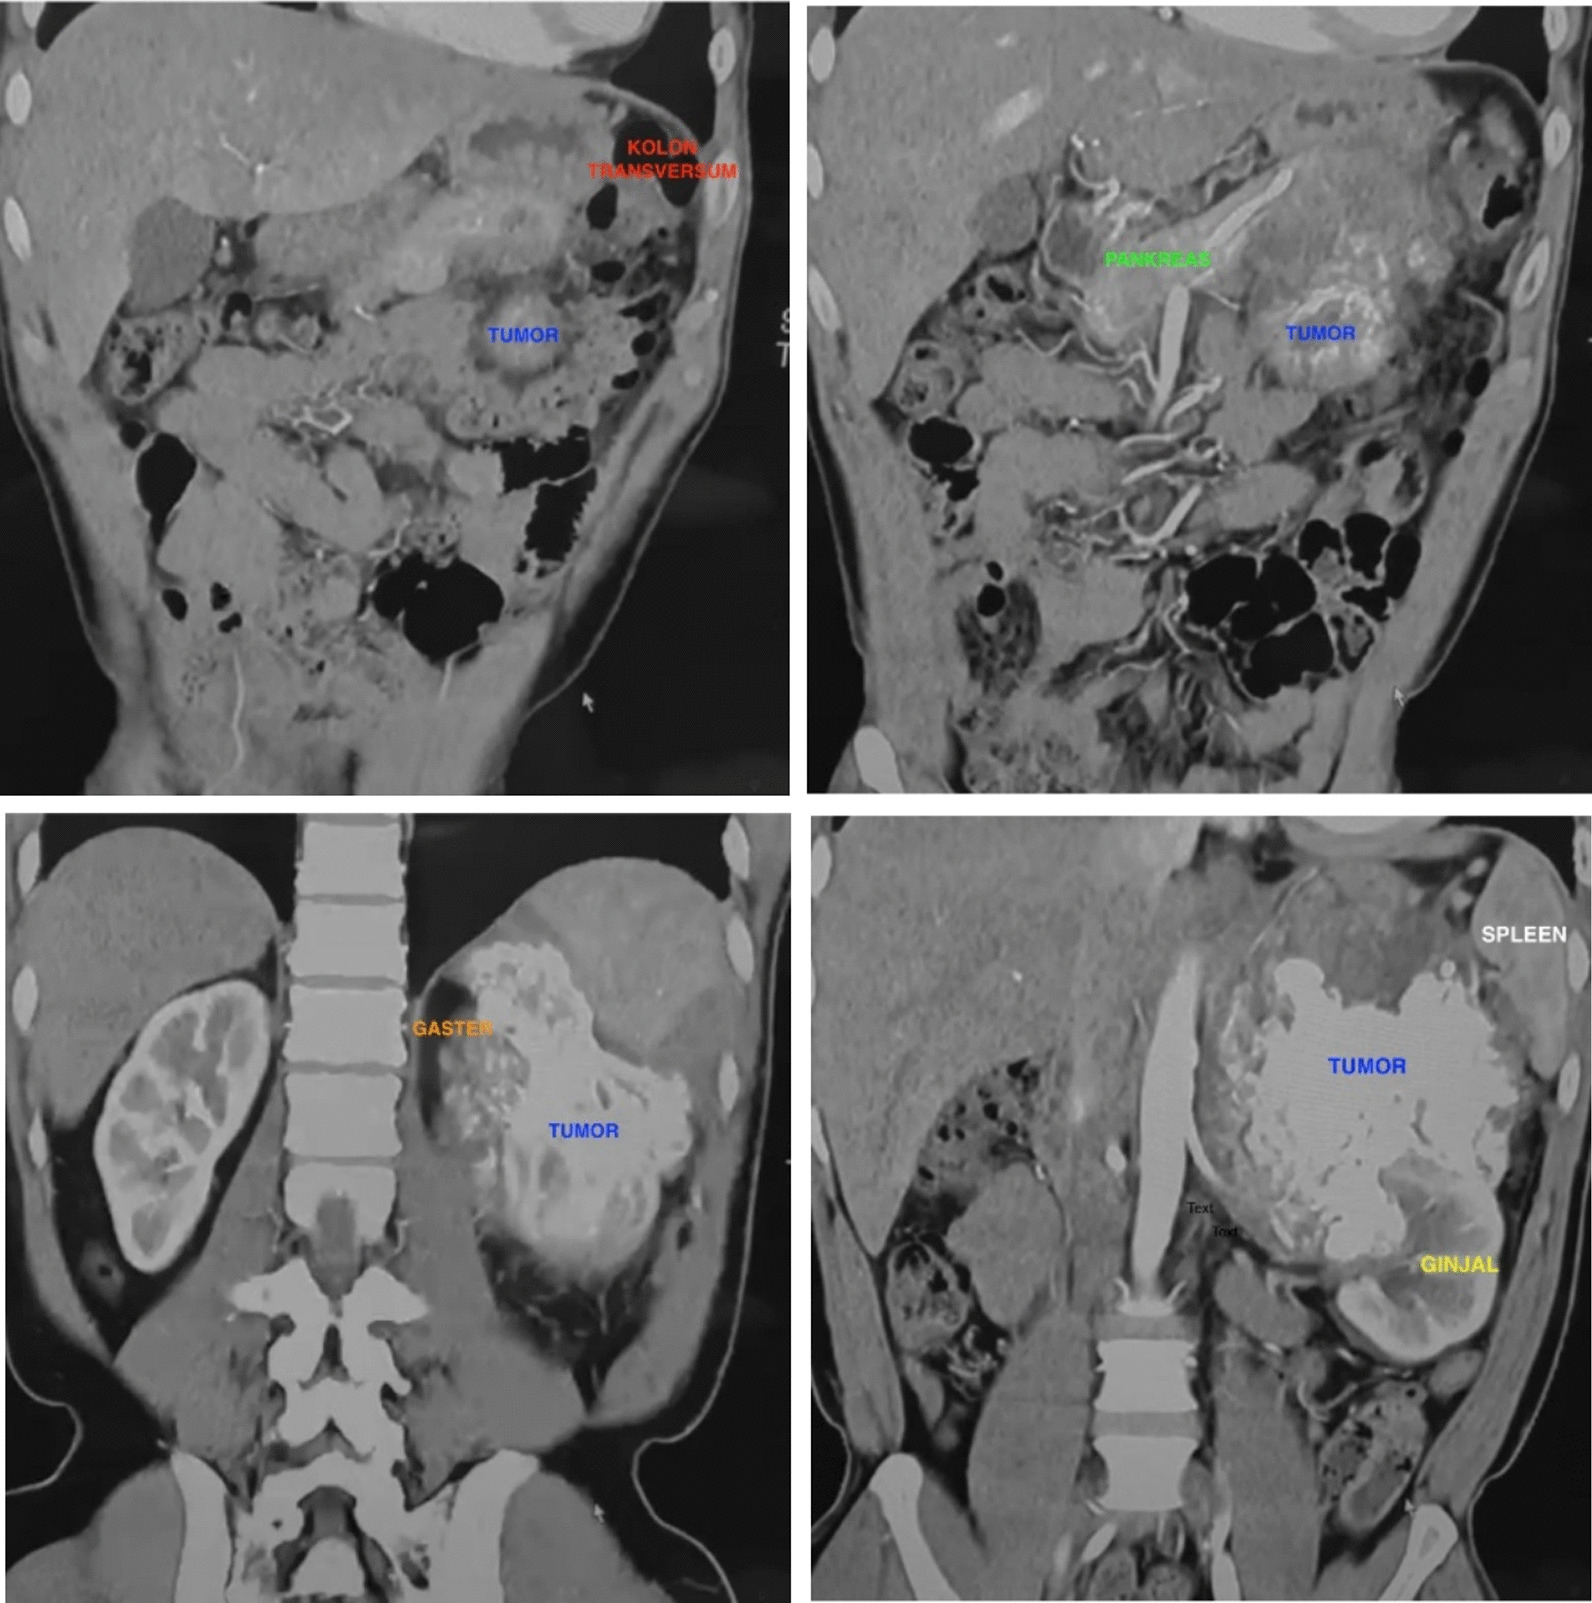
First computed tomography urography with contrast
